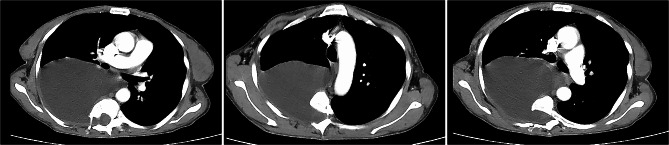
Chest CT scan revealed a large low-density mass in the right thoracic cavity that extended into the spinal canal. Scoliosis deformity in the thoracic spine, a defect on the right side of the T3-T5 thoracic vertebrae, and enlargement of the spinal canal and intervertebral foramen
